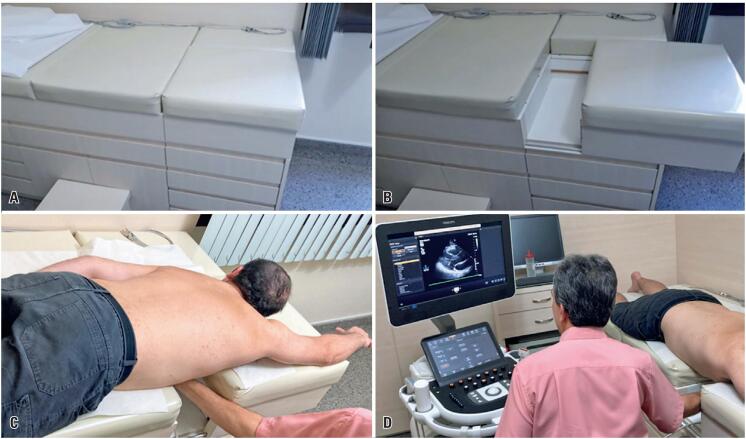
Adapted ultrasound table with an opening to allow access to the precordial region. (A) Table closed. (B) Table open. (C) Patient in the prone swimmer's position, with the right upper limb elevated. (D) Examiner's position for image acquisition
